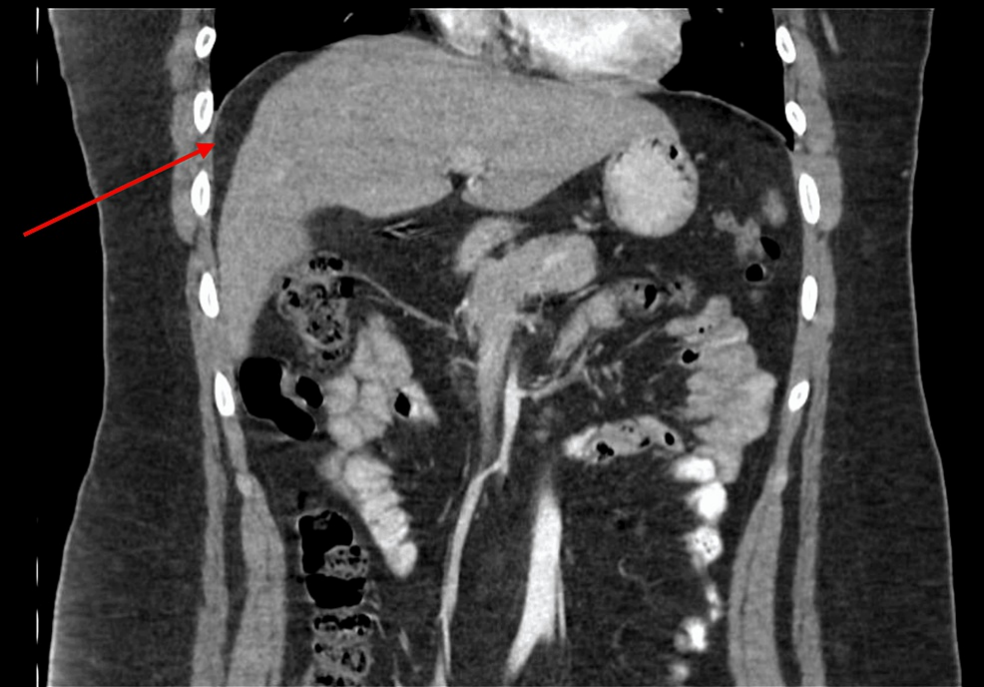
Coronal CT of Colonic Interposition (Red Arrow)
